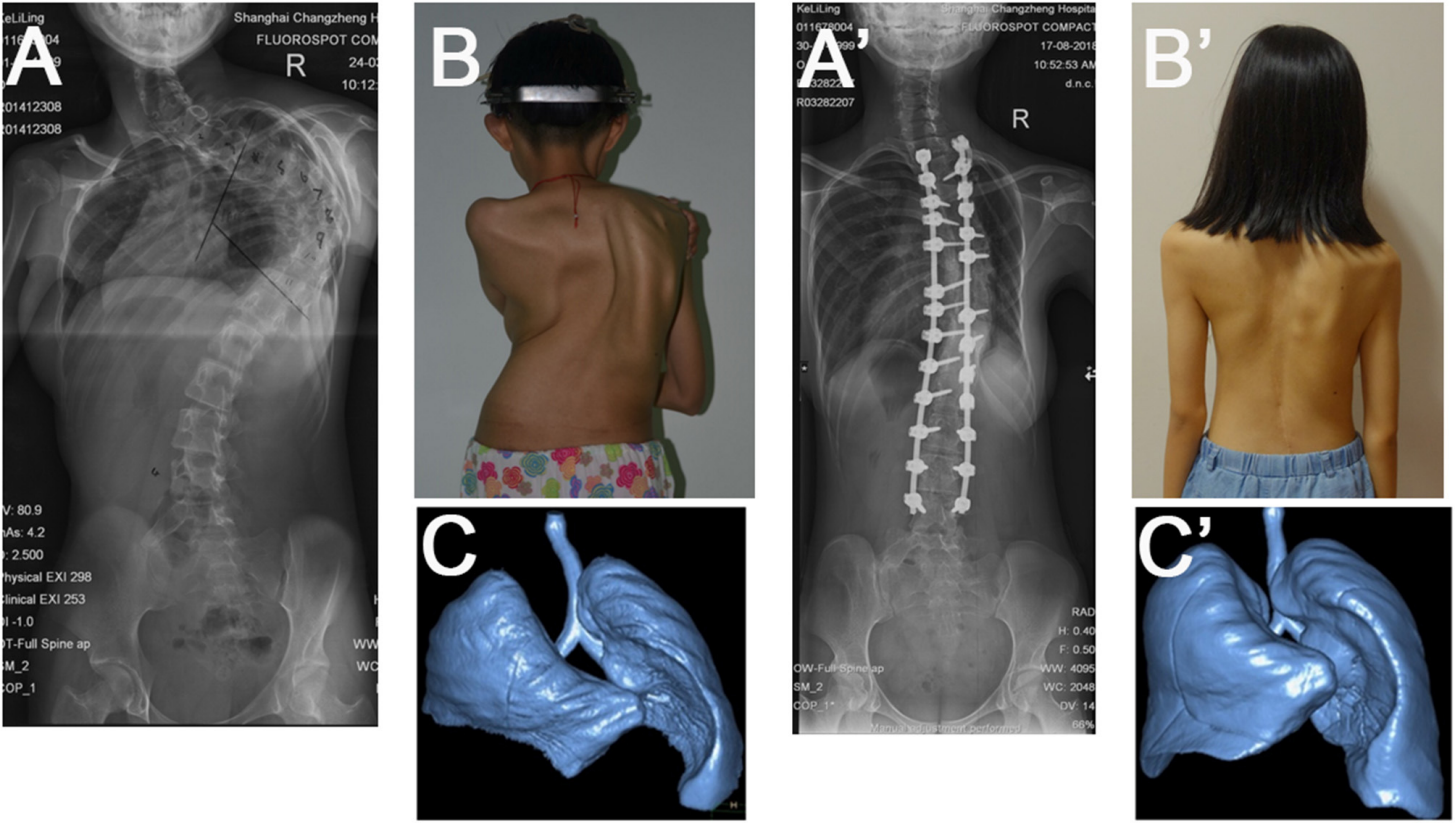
A 20-year-old female with early-onset idiopathic scoliosis. The initial Cobb angle was 112° (A,B). Halo gravity traction and posterior correction with instrumentation were performed. At 5-year follow-up, the scoliosis had decreased to 45° (A',B'). Improvement in the lung volumes was noted when compare preoperative reconstructed computed tomographic scan of the lungs (C) with ones at the last follow-up (C').
